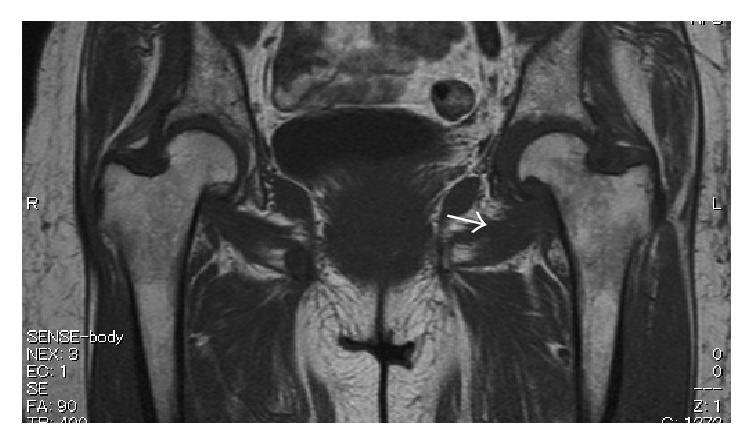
T1-weighted coronal magnetic resonance image at 1 month after surgery. There was no sign of avascular necrosis of the femoral head. The herniation sac had diminished in size (arrow).
